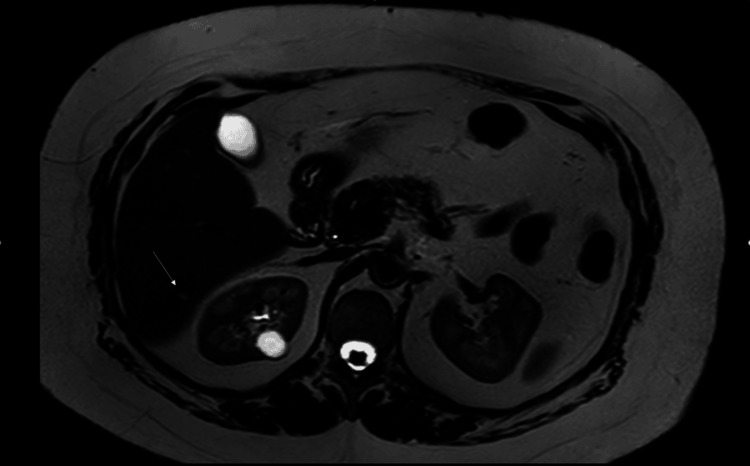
Abdominal MRI (HAVM). STIR abdominal MRI shows an early enhancing lesion in segment VII of the right liver lobe (white arrow), measuring about 1.6 cm, the appearance of which is suggestive of a small arteriovenous malformation. HAVM, hepatic arteriovenous malformation; STIR, short-TI inversion recovery
